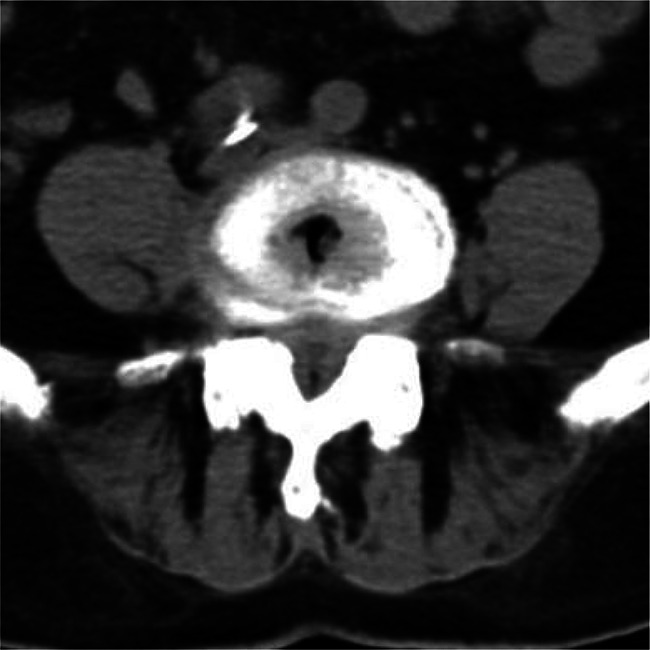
Preoperative computed tomography (CT) examination confirmed the diagnosis of spinal stenosis.
